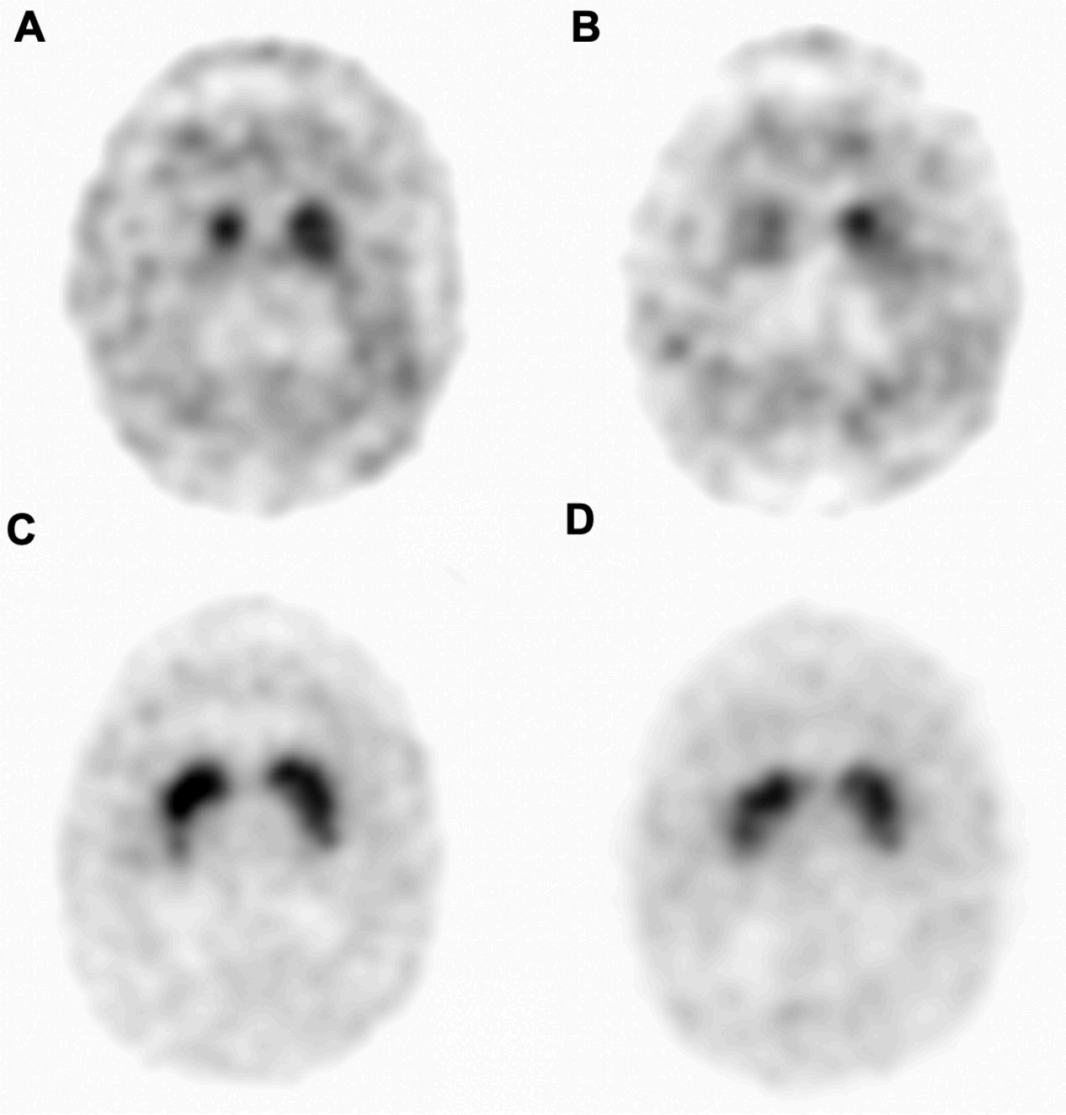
Representative cases of a PD and PD-SWEDD patients. I-123 FP-CIT SPECT images of a PD (A, B) and PD-SWEDD (C, D) patients at baseline (A, C) and at 2-year follow-up (B, D). Baseline MDS-UPDRS III scores were 36 for both patients. 2-year MDS-UPDRS III scores were 59 for the PD patient, and 61 for the PD-SWEDD patient.
